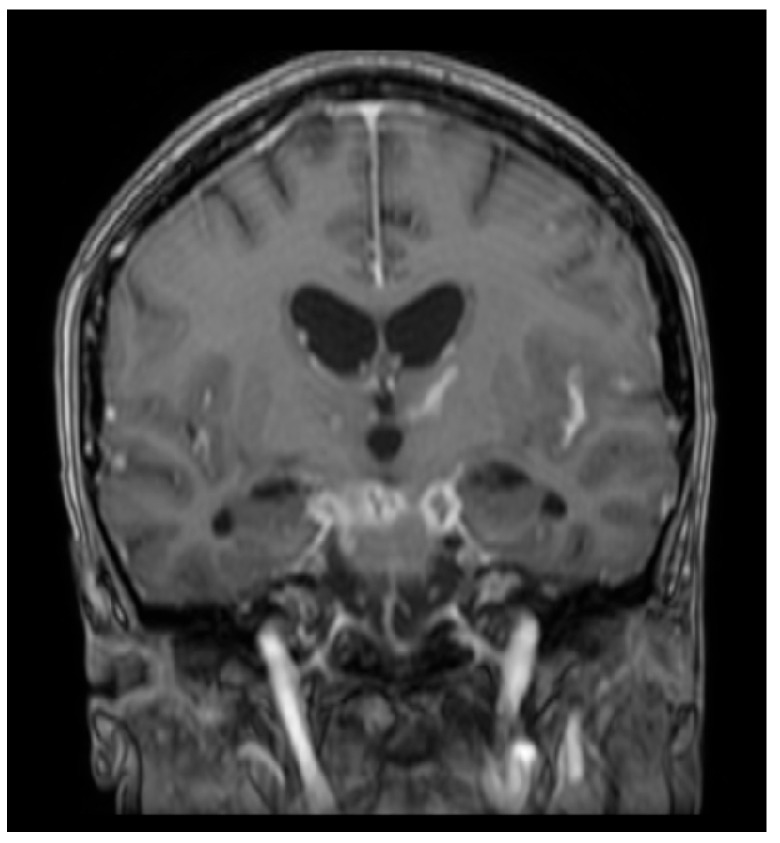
Post-contrast T1-weighted MRI of a patient with tuberculous meningitis demonstrating marked leptomeningeal enhancements and enhancing basal exudates. Case courtesy of Ammar Haouimi, Radiopaedia.org, rID: 72617, licensed under CC-NC-BY-SA 3.0 [27].
